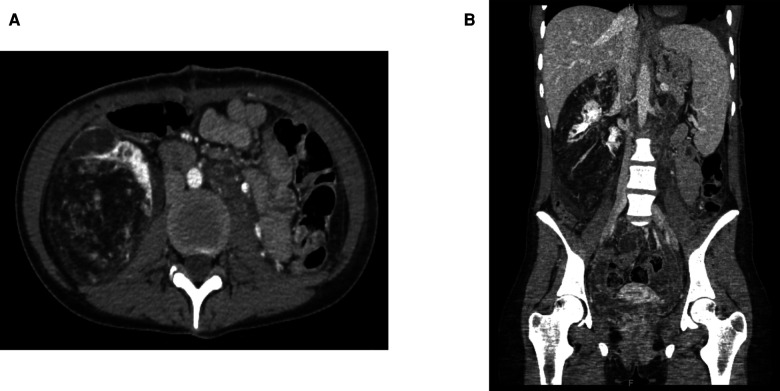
Postoperative contrast-enhanced computed tomography of the abdomen: (A) axial plane; (B) sagittal plane.
